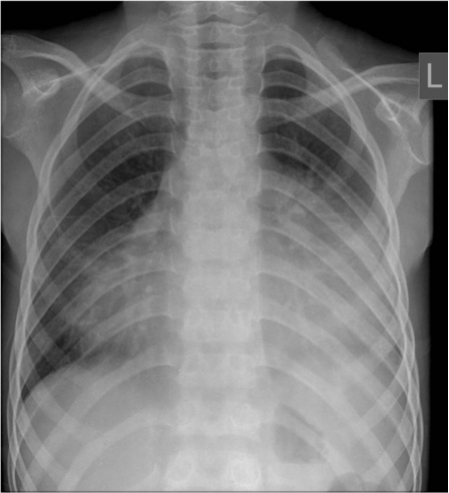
Posteroanterior chest radiograph at presentation revealed a markedly enlarged cardiac silhouette and a left-sided pulmonary infiltrate.
